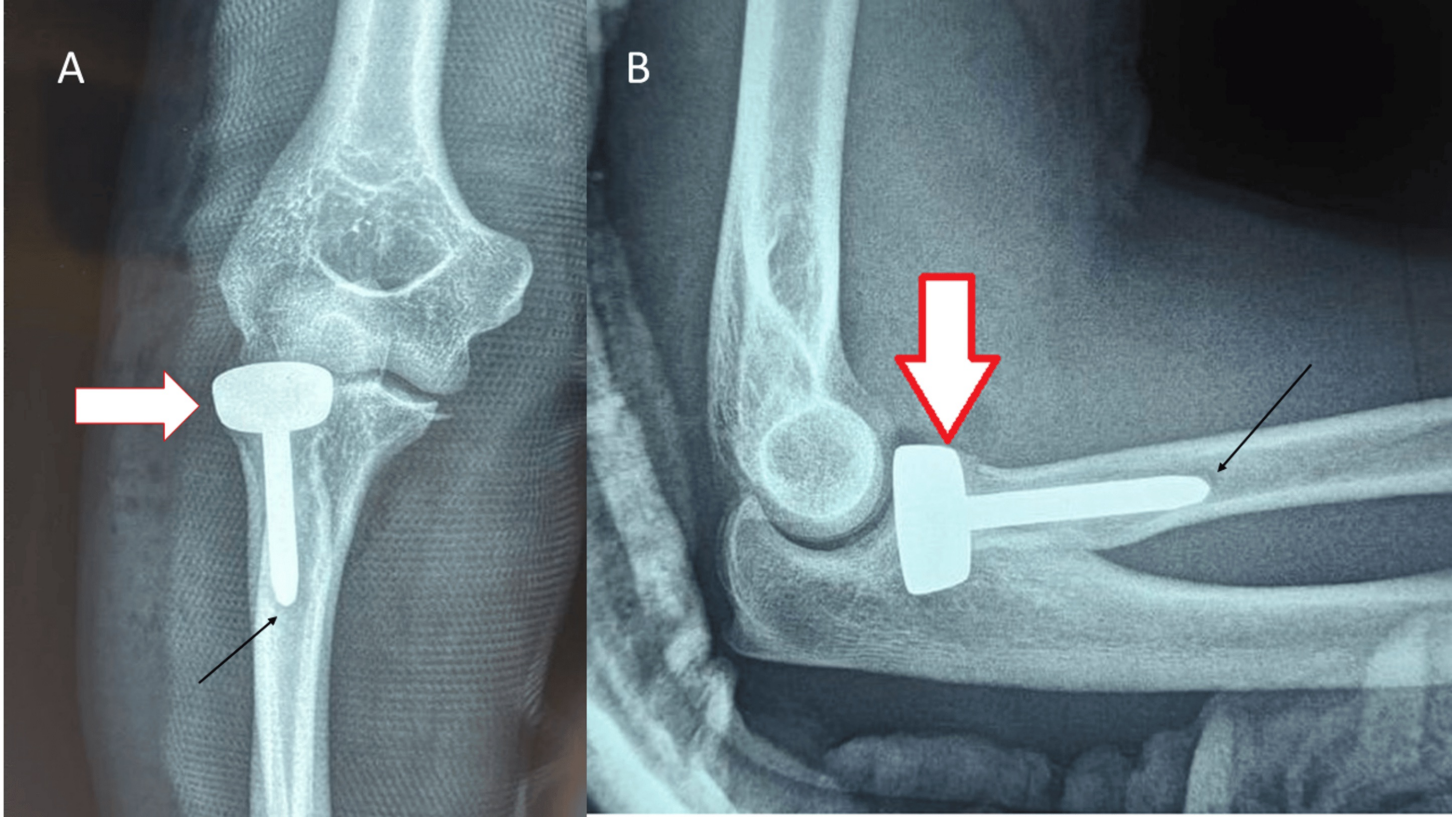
A) Post-operative radiograph showing the anteroposterior (AP) view of the right elbow with Mason type III radial head fracture. B) Post-operative radiograph showing the lateral view of the right elbow with Mason type III radial head fracture. The white arrow indicates the radial head prosthesis, while the black arrow highlights the cement mantle surrounding the implant.
